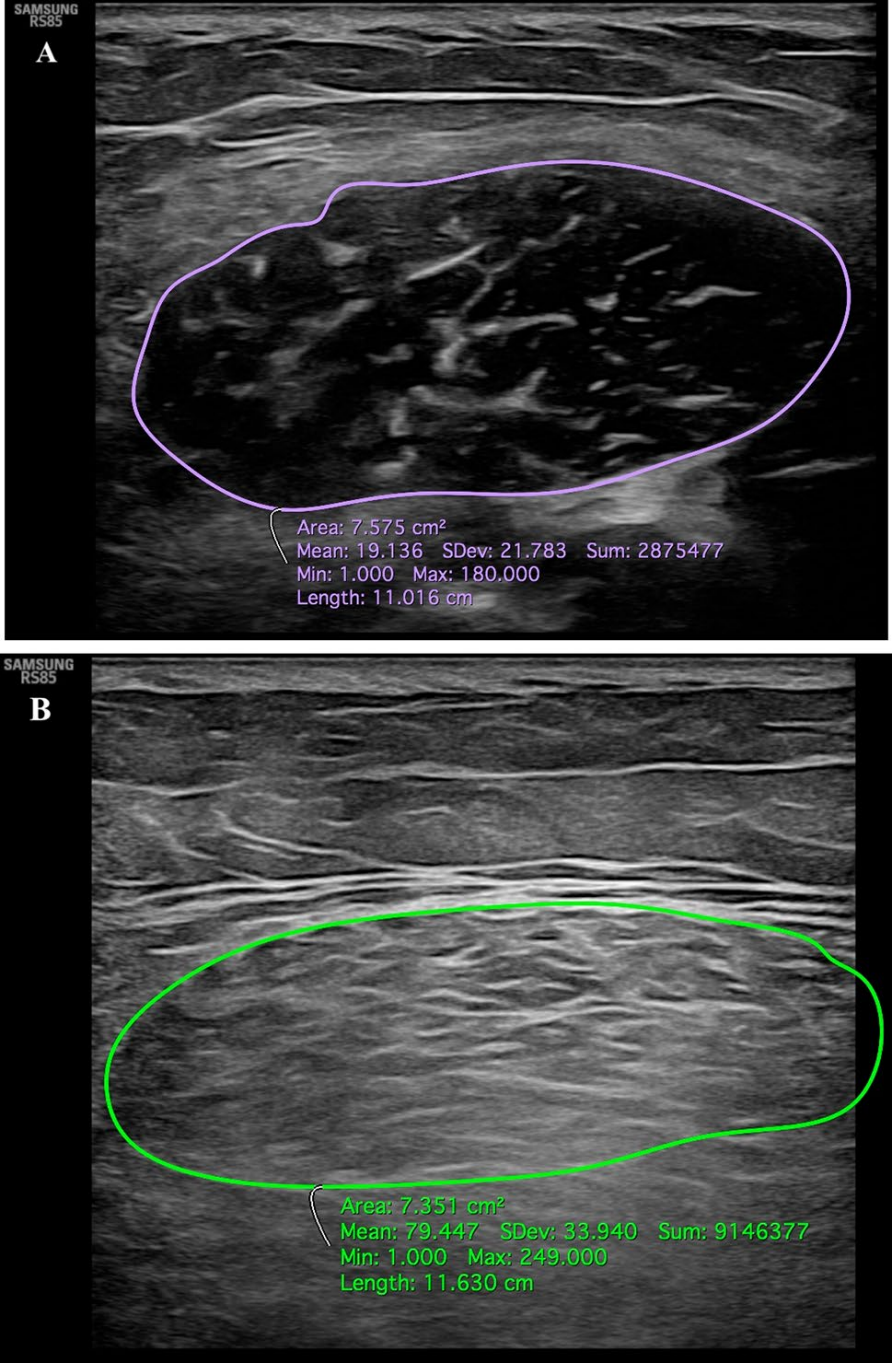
Ultrasound transverse images of the proximal third of the rectus femoris of two female patients with similar age and BMI. Image A shows the rectus femoris of 38-year-old runner (BMI = 24.2), while image B shows the rectus anterior of a 42-year-old patient (BMI = 25.1) who does not practice any physical activity. Despite a similar muscle trophism (CSA of patient in image B = 7.575 cm^2^, CSA of patient in image B = 7.351 cm^2^), an increased muscle belly echogenicity can be observed in the patient with limited physical activity due to fatty infiltration (image B)
